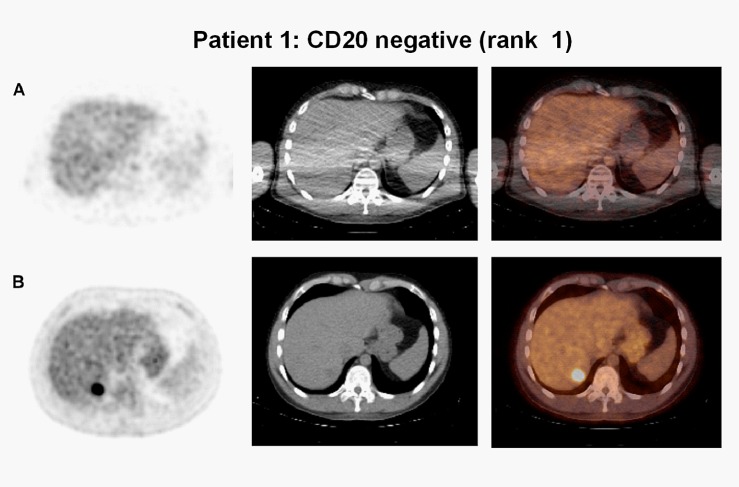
No tumor uptake on ^89^Zr-rituximab-PET, concordant with CD20 expression in biopsy. Axial images, from left to right attenuation corrected PET, low dose CT and fused PET/CT image of patient 1. a) ^89^Zr-rituximab-PET shows no tumor uptake concordant with a CD20-negative liver biopsy. b) Corresponding tumor location on ^18^F-FDG-PET.
